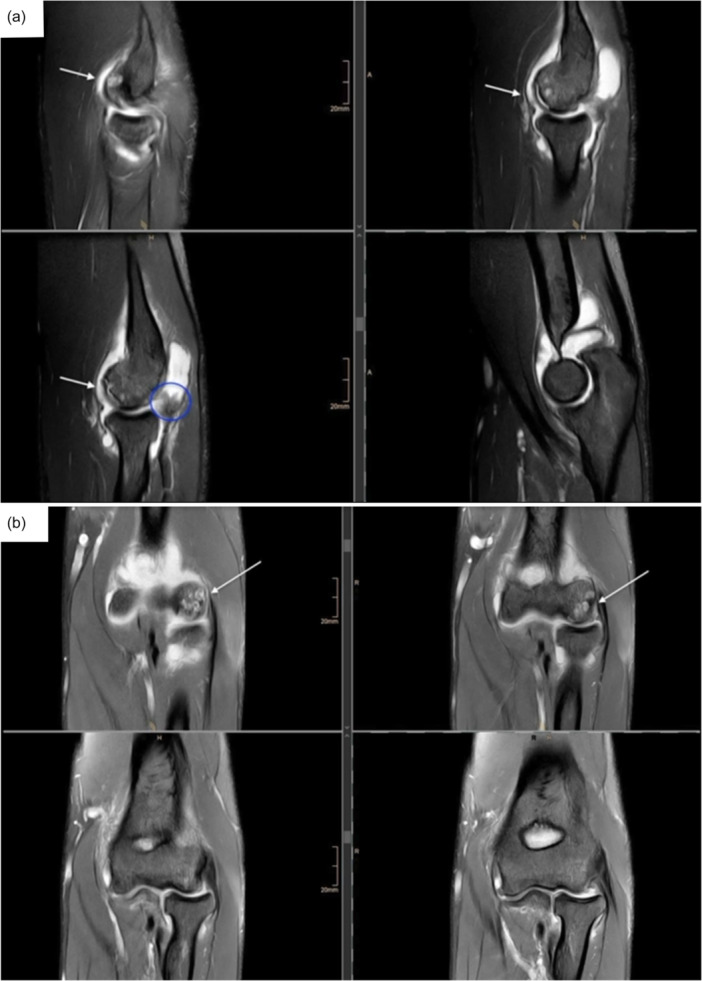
Preoperative elbow MRI of a representative patient with osteochondrosis dissecans. Representative preoperative MRI of the elbow in the sagittal (a) and coronar plane (b) demonstrating a focal osteochondral defect of the capitellum, marked by an arrow. In addition, hypertrophic tissue is visible in the region of the posterolateral plica, highlighted by the blue circle. MRI, magnetic resonance imaging.
